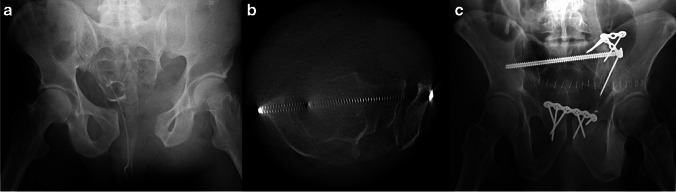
A Pre-operative AP radiograph demonstrating APC III pelvis injury to a patient after a motorcycle accident. B intra-operative O-arm scan demonstrated mal-reduction to the left sacroiliac joint as well as an incarcerated bone fragment. C Post-operative pelvic inlet radiograph demonstrating final fixation of pelvic ring injury
